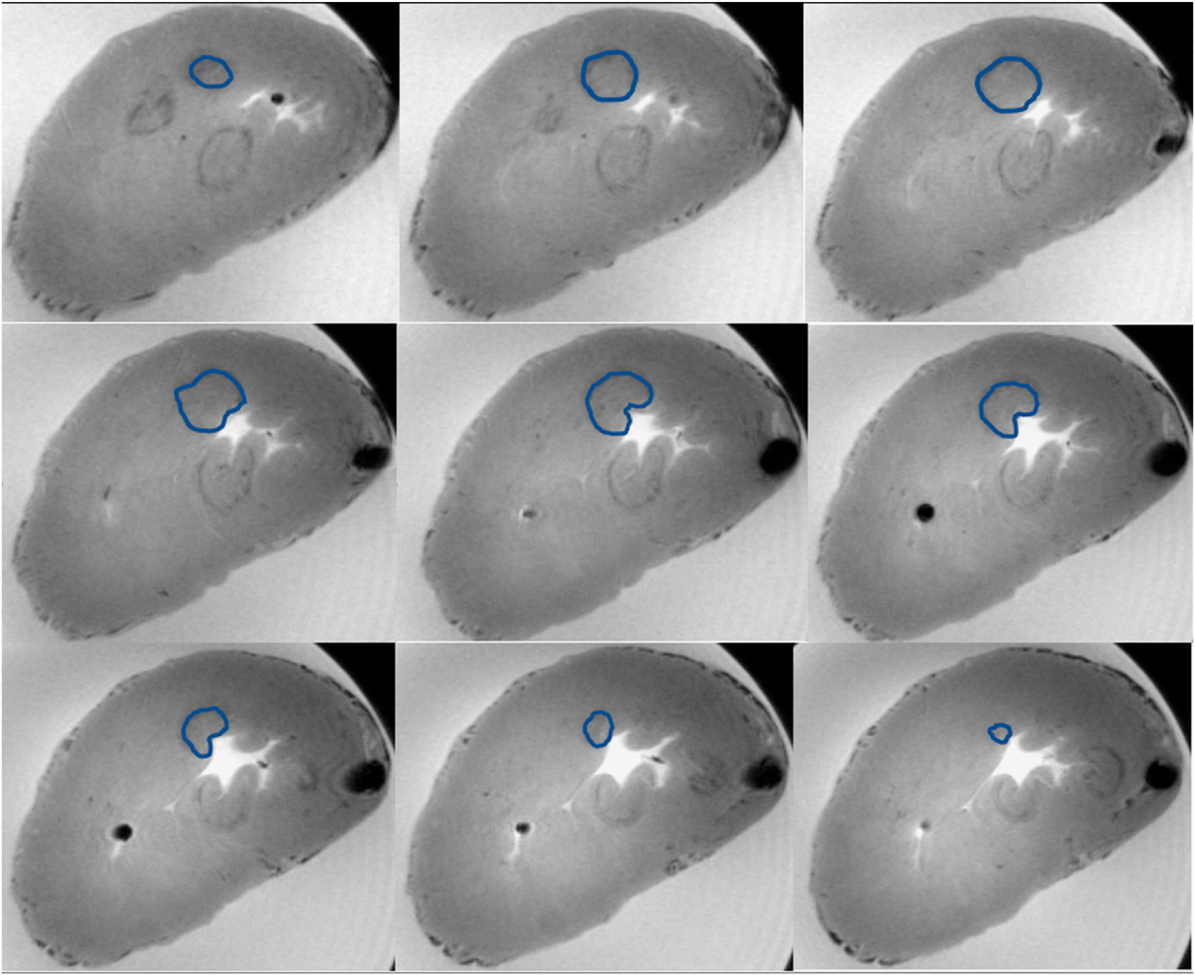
Example of volume measurement with the “point-by-point” method from MRI data. On the picture you can see nine subsequent transverse sections of the left ventricle capturing the whole radiofrequency lesion. Three lesions are visible, the upper one is measured. The measured lesion is outlined in all scans with a blue line. The resulting volume is the sum of the individual volumes from all involved MRI scans.
